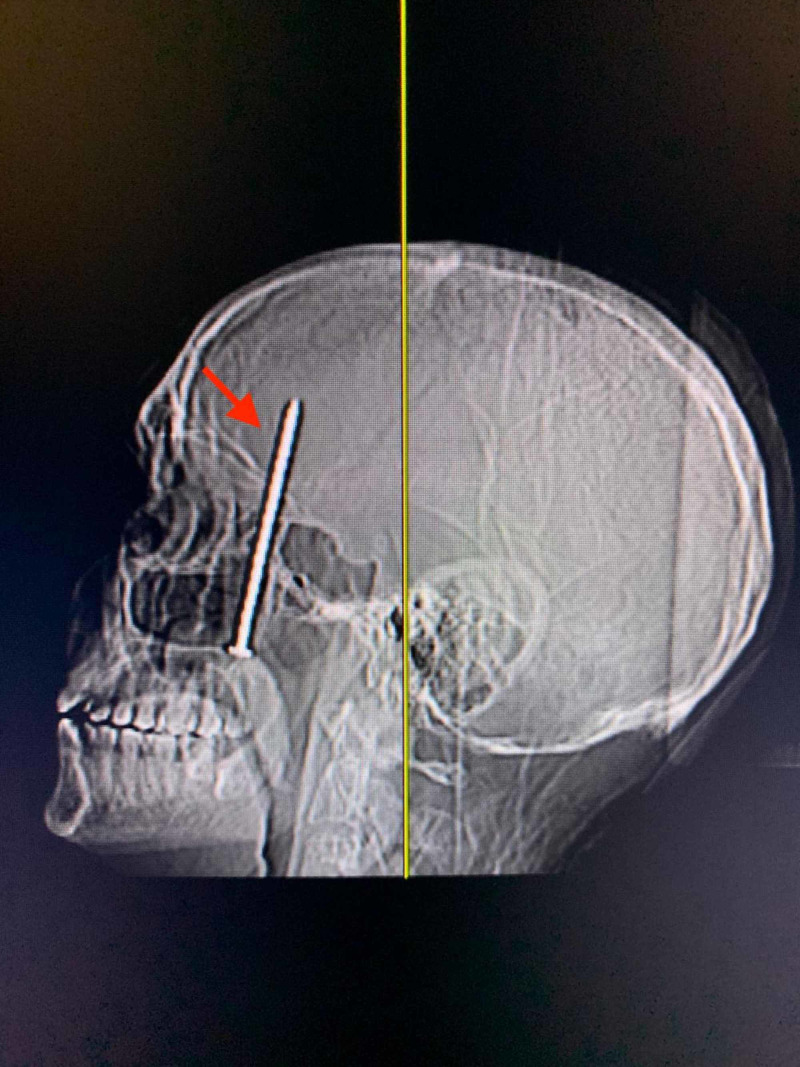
Lateral X-ray of the skull demonstrating penetration of the anterior skull base
